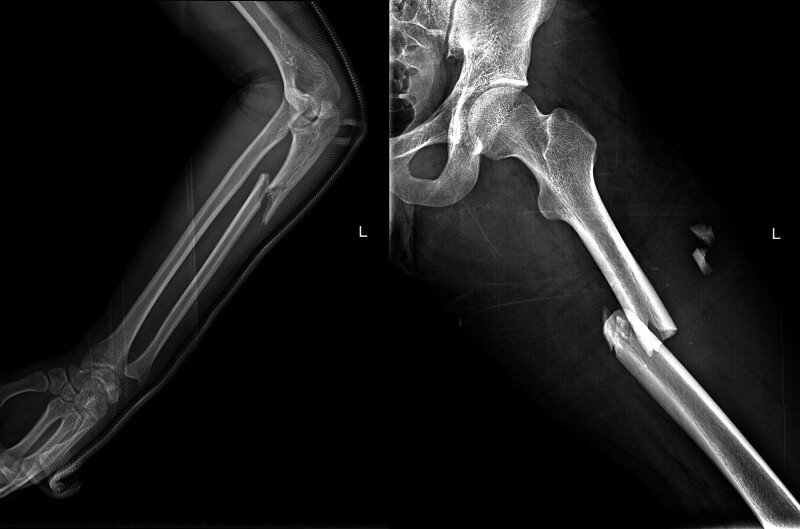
X-ray showed the fracture of the left proximal ulna (left) and the left femoral shaft (right).
